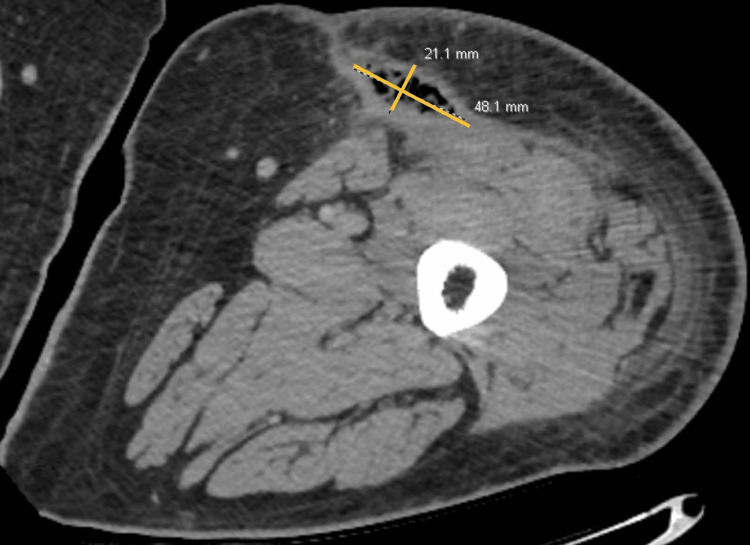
Post-debridement CT left lower extremity shows 2 x 4.8 cm focus of gas contained within the anteromedial thigh.
